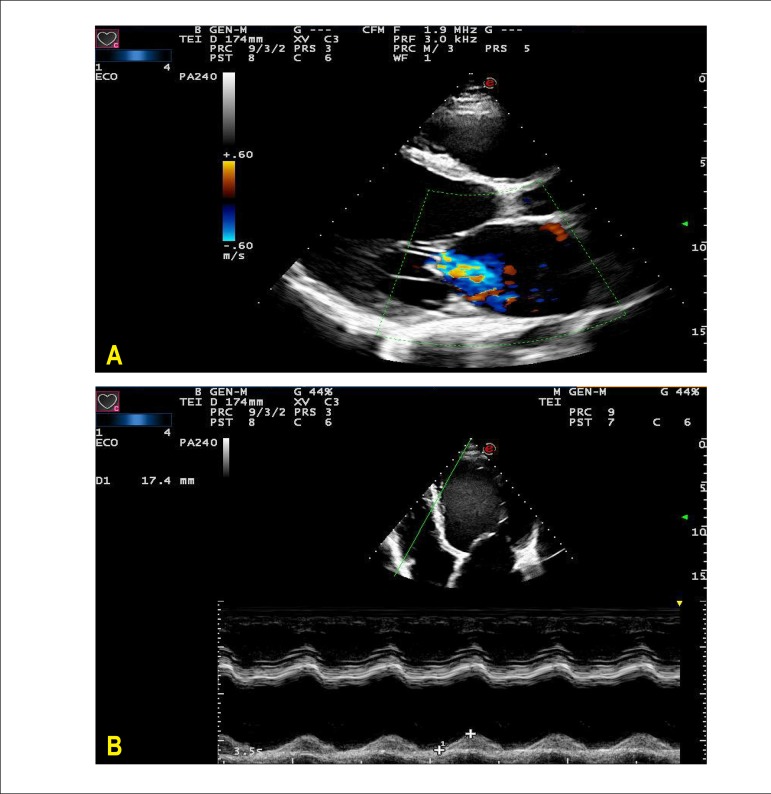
Echocardiogram. A) Longitudinal parasternal view with Doppler. Severe mitral
